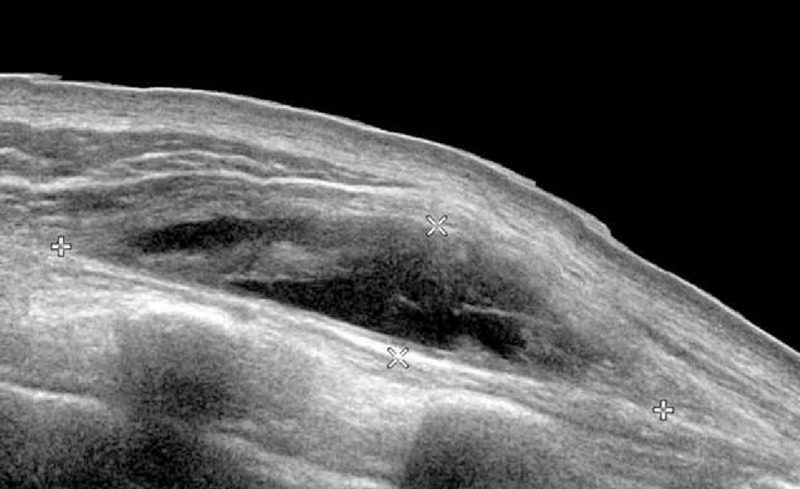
Longitudinal view of left shoulder on ultrasonography and periscapular area shows heterogenous fluid-filled cystic mass.
